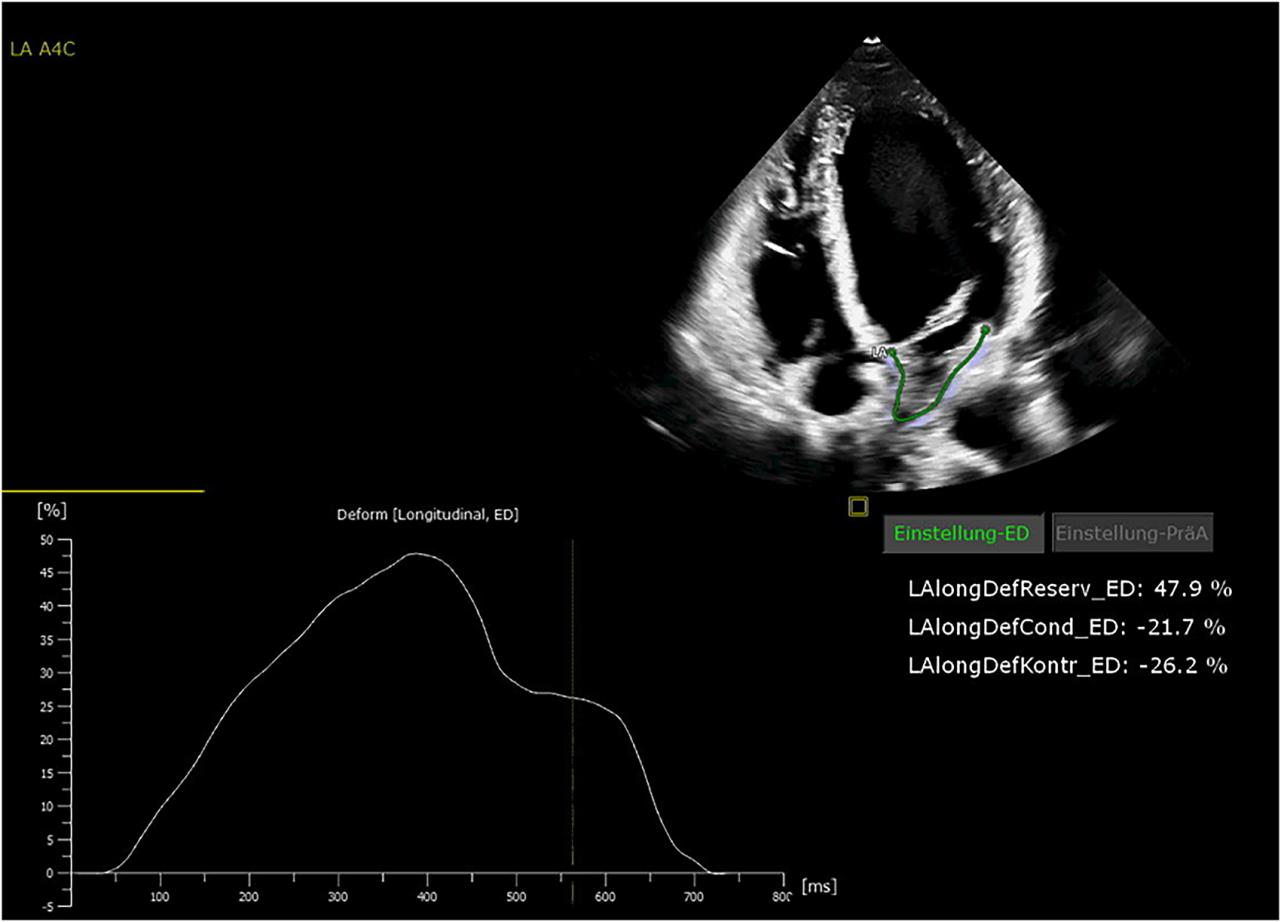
Echocardiography showing normal left atrial (LA) strain values.
